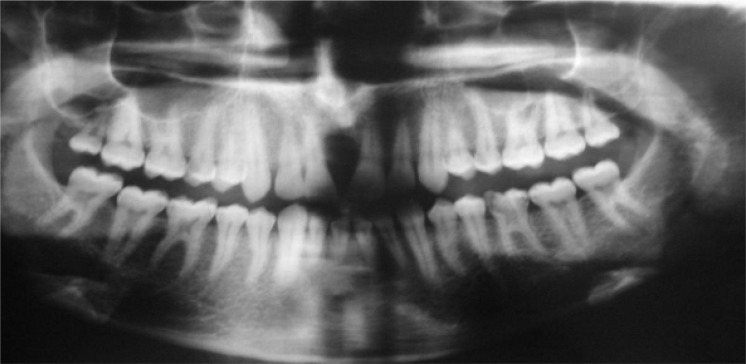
Panoramic radiograph showing a unilocular radiolucent area between central incisors. Note the roots of two central incisors are displaced distally
